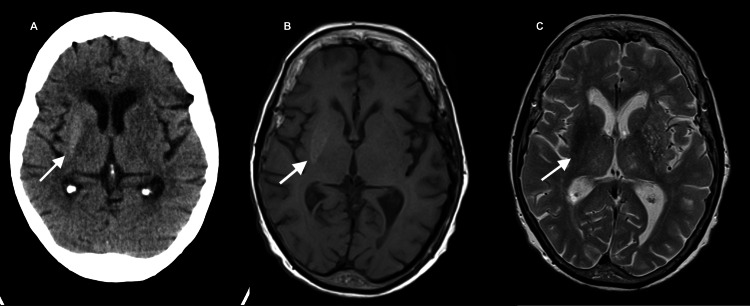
Image study of case 1. A. Head CT scan showing lenticular and right capsular hyperdensity. B. Head MRI, T1, showing hypersignal in the lenticular right nucleus. C. Head MRI, T2, showing hyposignal of the lenticular right nucleus.
